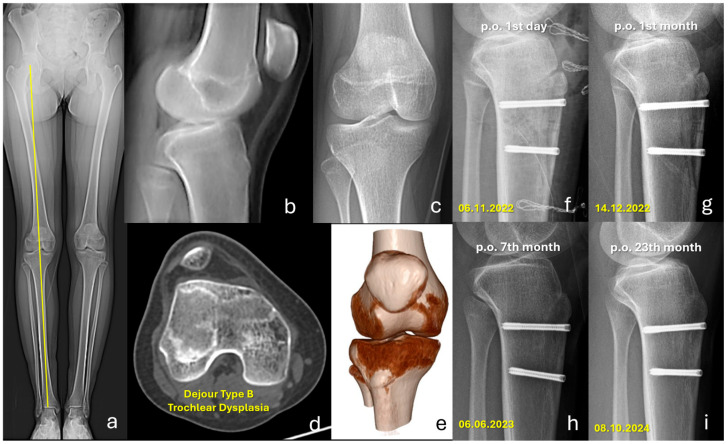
Preoperative imaging and postoperative follow-up of a 13-year-old female who presented with recurrent patellar dislocation. (a) Preoperative standing full-length anteroposterior radiograph demonstrating normal lower-limb alignment (yellow line indicates the mechanical axis). (b,c) Preoperative lateral and anteroposterior knee radiographs. (d) Axial computed tomography (CT) image showing a flat trochlea, consistent with Dejour type B trochlear dysplasia. (e) Three-dimensional CT reconstruction demonstrating lateralization of the tibial tubercle with an increased tibial tubercle–trochlear groove (TT–TG) distance (22.5 mm). (f) Postoperative day 1 radiograph after tibial tubercle osteotomy, fixed with two headless compression screws. (g–i) Follow-up radiographs at 1, 7, and 23 months postoperatively showing progressive healing and union of the osteotomy site.
